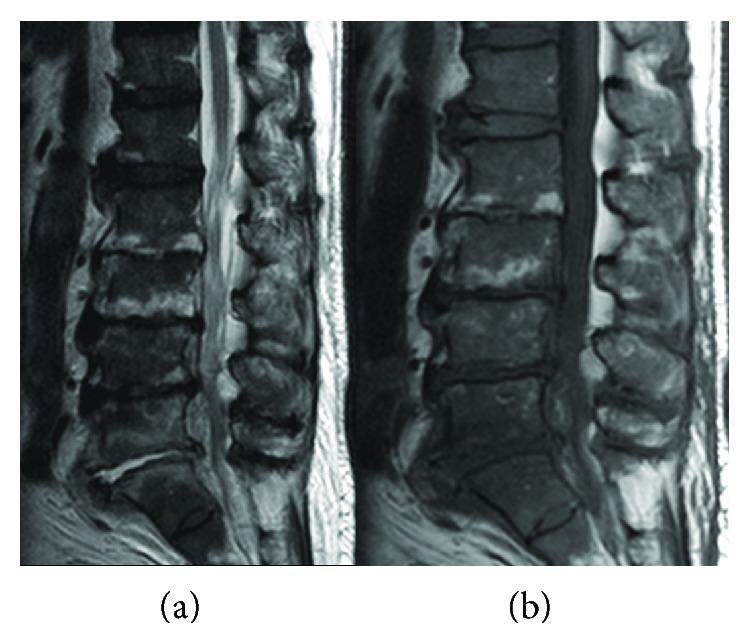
Magnetic resonance images of the lumbar spine. High-intensity lesion at the L5/S disc without epidural abscess was detected on a T2-weighted image (a). A low-intensity lesion at the same level was detected on a T1-weighted image (b).
